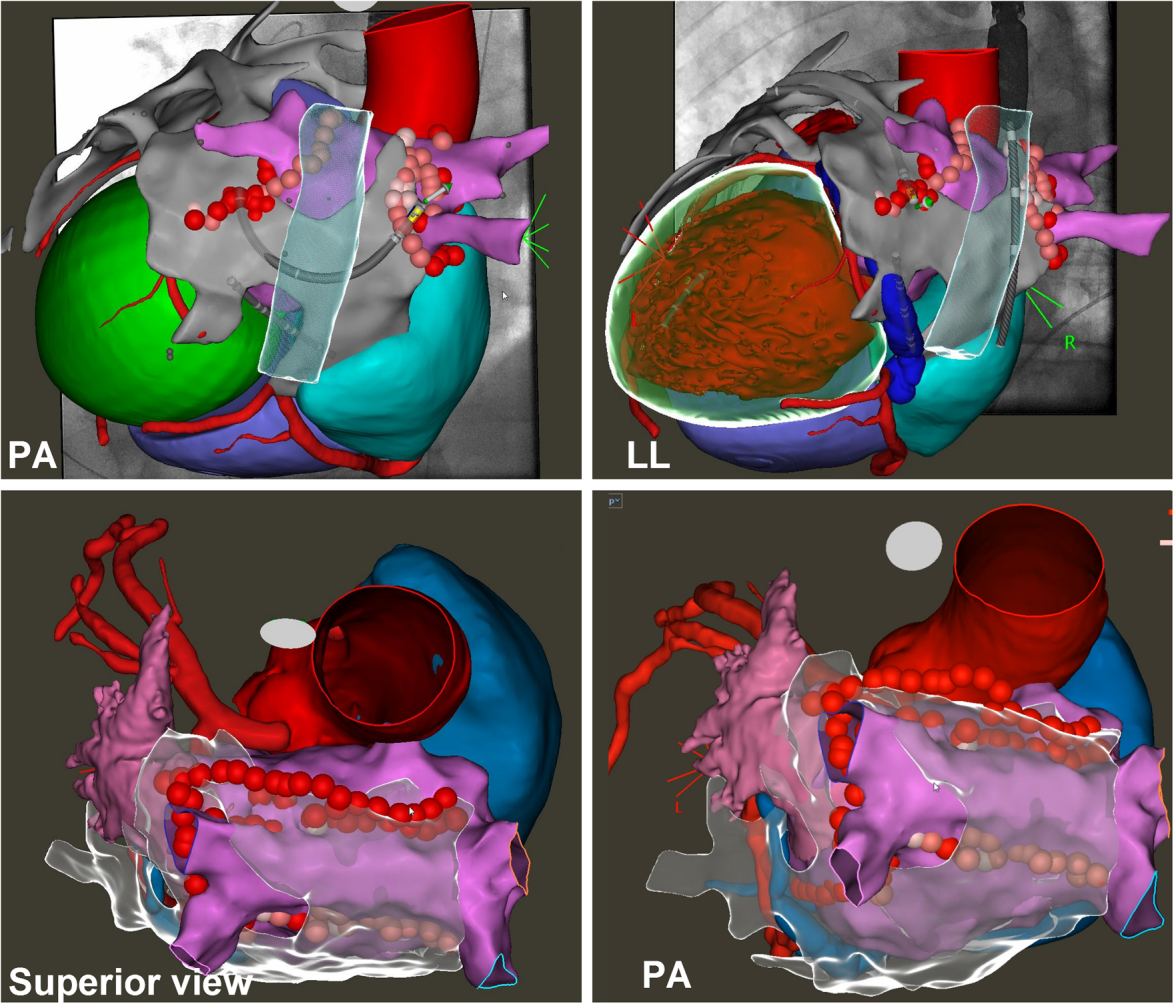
Endo-epicardial atrial fibrillation ablation. The images show the 3D electroanatomical left atrium (pink) and epicardium (grey) maps synchronized with the CT scan and fluoroscopic images. On the maps, it is possible to see the ablation lines performed both endocardially (PVI, roof line, and posterior line) and epicardially (PVI, roof line, and left isthmus line). See text.
